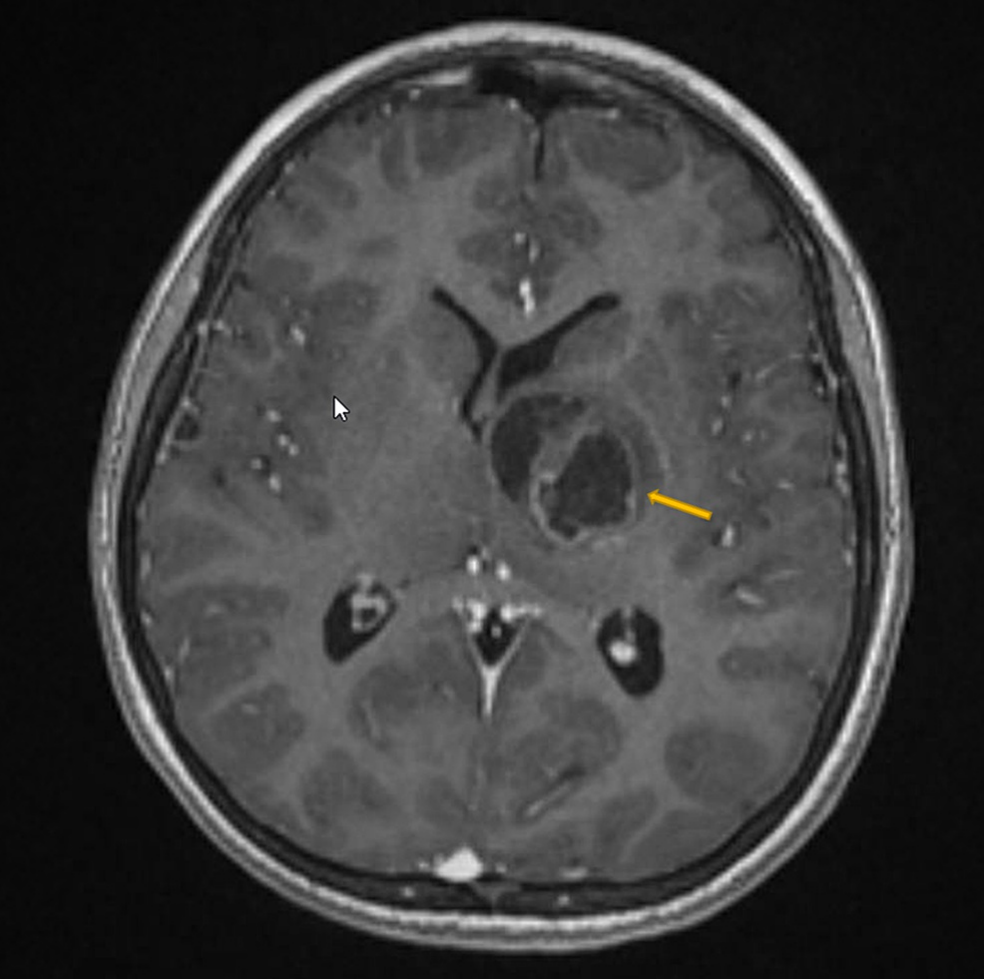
Preoperative axial T1-weighted MRI with contrast The image shows a left thalamic lesion, a heterogeneous mass with internal necrosis, and hemorrhagic components centered within the left thalamus with involvement of the left cerebral peduncle and basis of the midbrain, suggestive of high-grade glioma.
